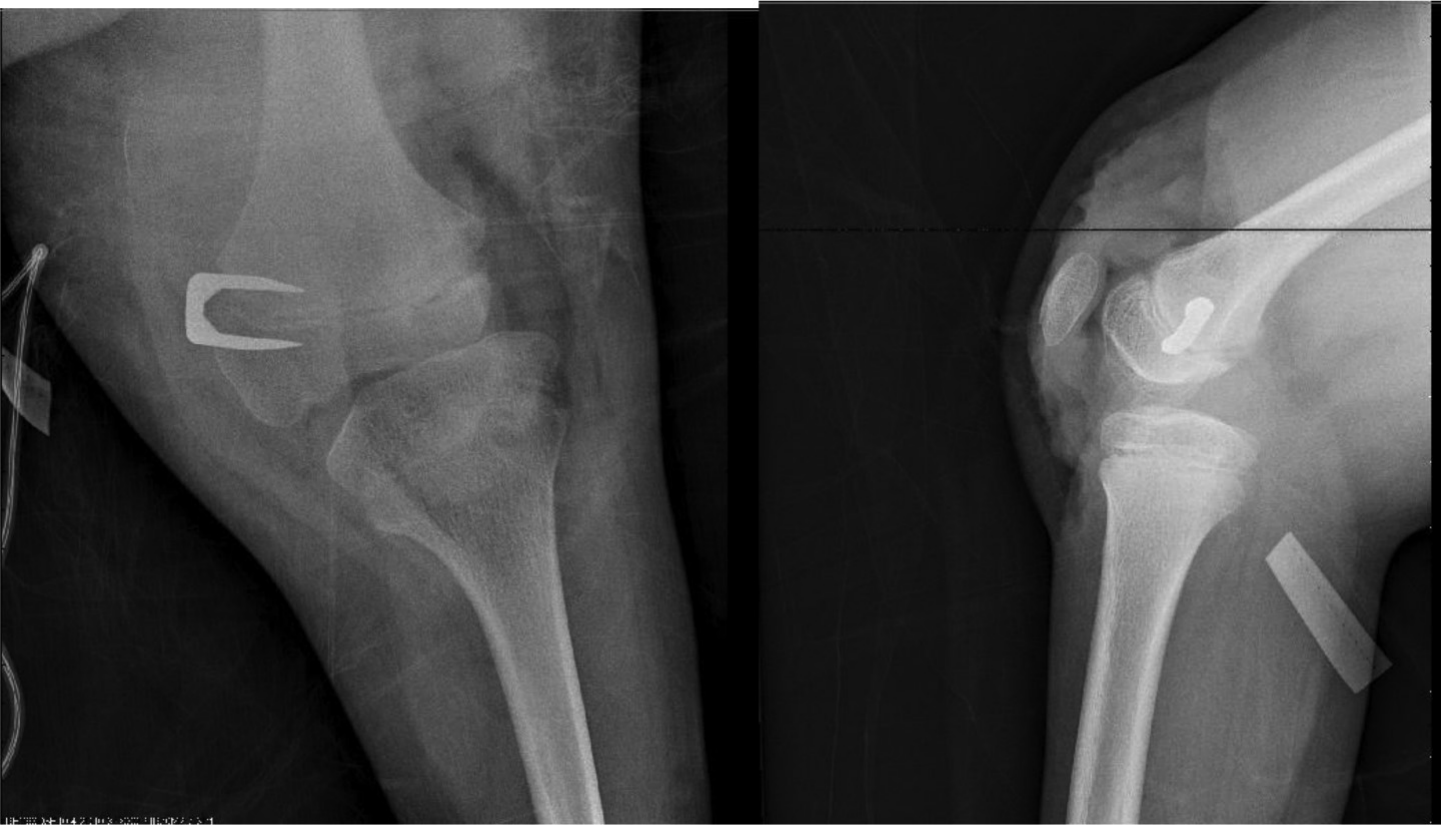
Anteroposterior and lateral radiographs of the left knee. Reduction of patellar luxation, using the modified Roux-Goldthwait technique, with medial temporary hemiepiphysiodesis to treat valgus.
